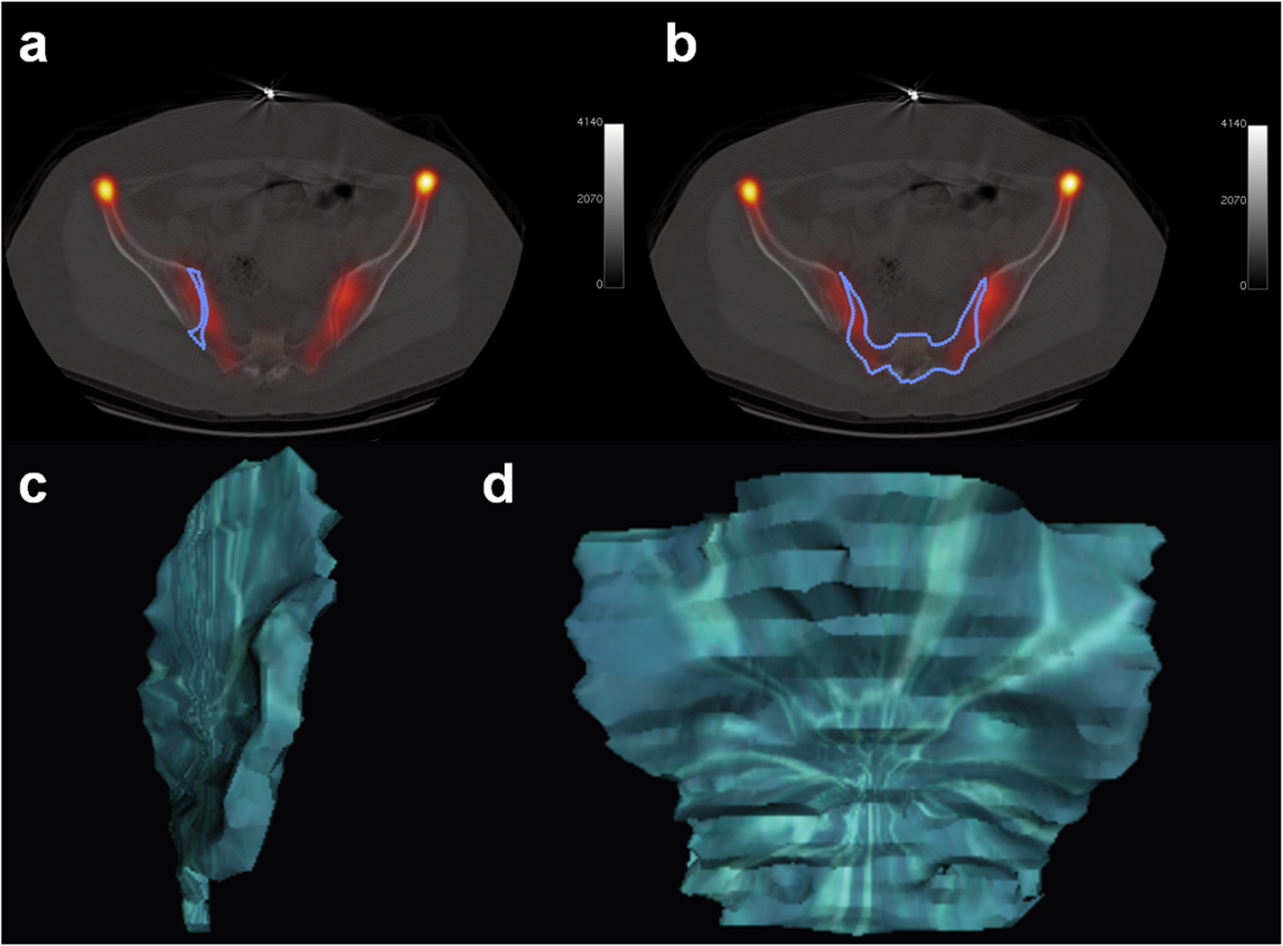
The volume of interest (VOI) of sacroiliac (SI) joint and sacrum for SI joint to sacrum (SIS) uptake ratio. The VOI images of SI joint (a) and sacrum (b) defined in transxial image of bone single positron emission computed tomography-computed tomography (SPECT/CT). Three-dimensional (3D) reconstructed VOI images of SI joint (c) and sacrum (d) used to calculate the SIS uptake ratio in bone SPECT/CT.
